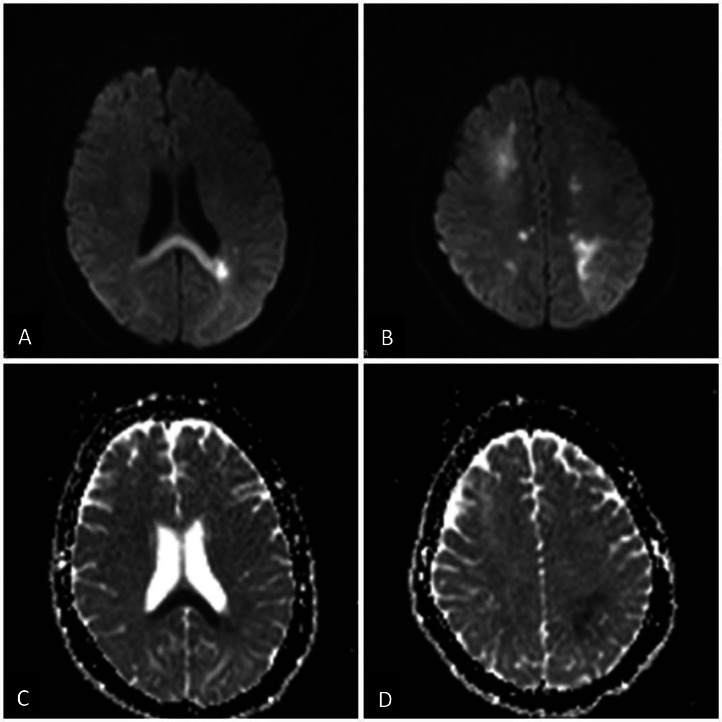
(A and B) DWI and (C and D) ADC maps images demonstrate true restriction in the corpus callosum and both centrum semiovale in favor of CLOCCs and multifocal acute infarction.
